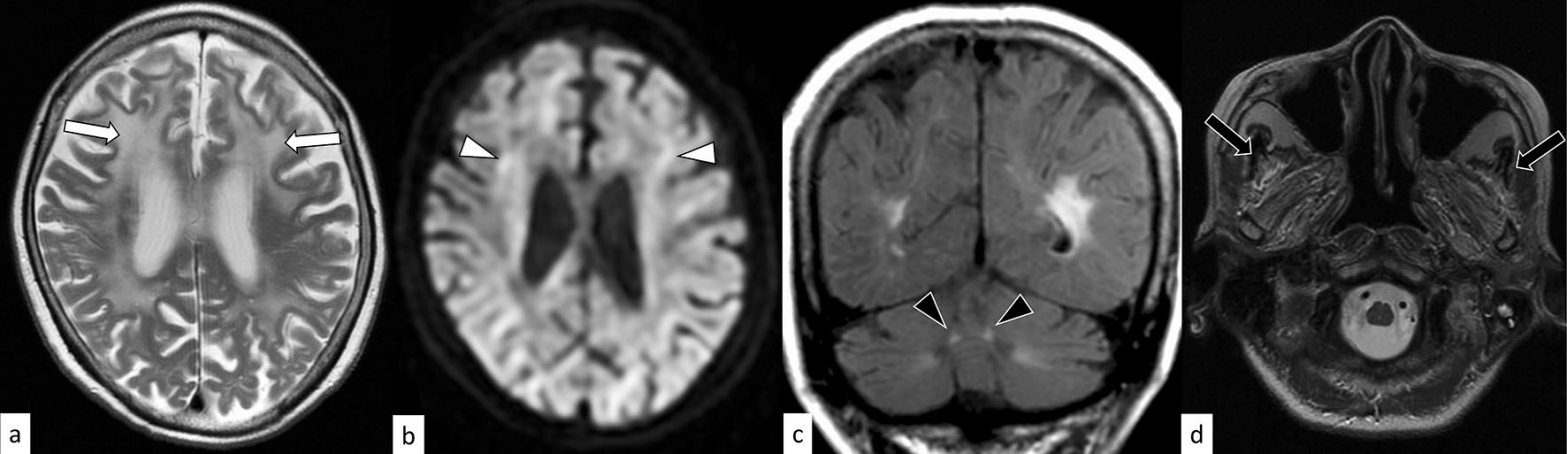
A 60-year-old woman with OPML. T2-weighted axial image shows hyperintensity in the white matter with atrophy (a, white arrows). DWI shows hyperintensity bands along the corticomedullary junction (b, white arrowheads). FLAIR coronal image also shows paravermal hyperintensity (c, black arrowheads). Atrophy and fat infiltration of the bilateral masticator muscles are observed on T2-weighted axial image (d, black arrows). The same case with different MRI slides was evaluated in a prior study [6]
